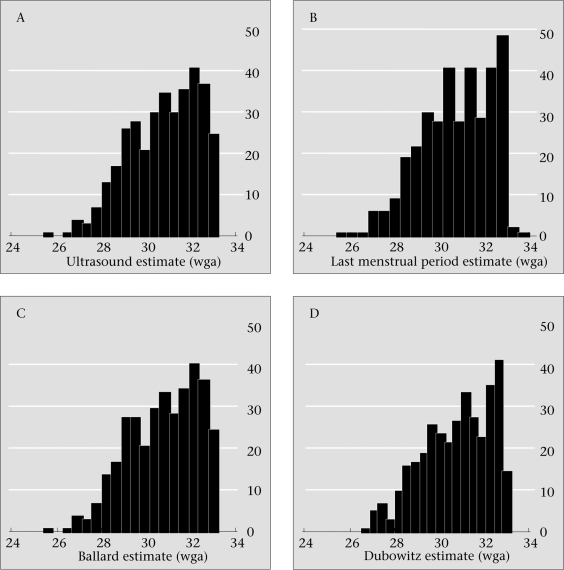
Distribution of estimates of gestational age by (A) ultrasound, (B) last menstrual period, (C) Ballard, and (D) Dubowitz.
